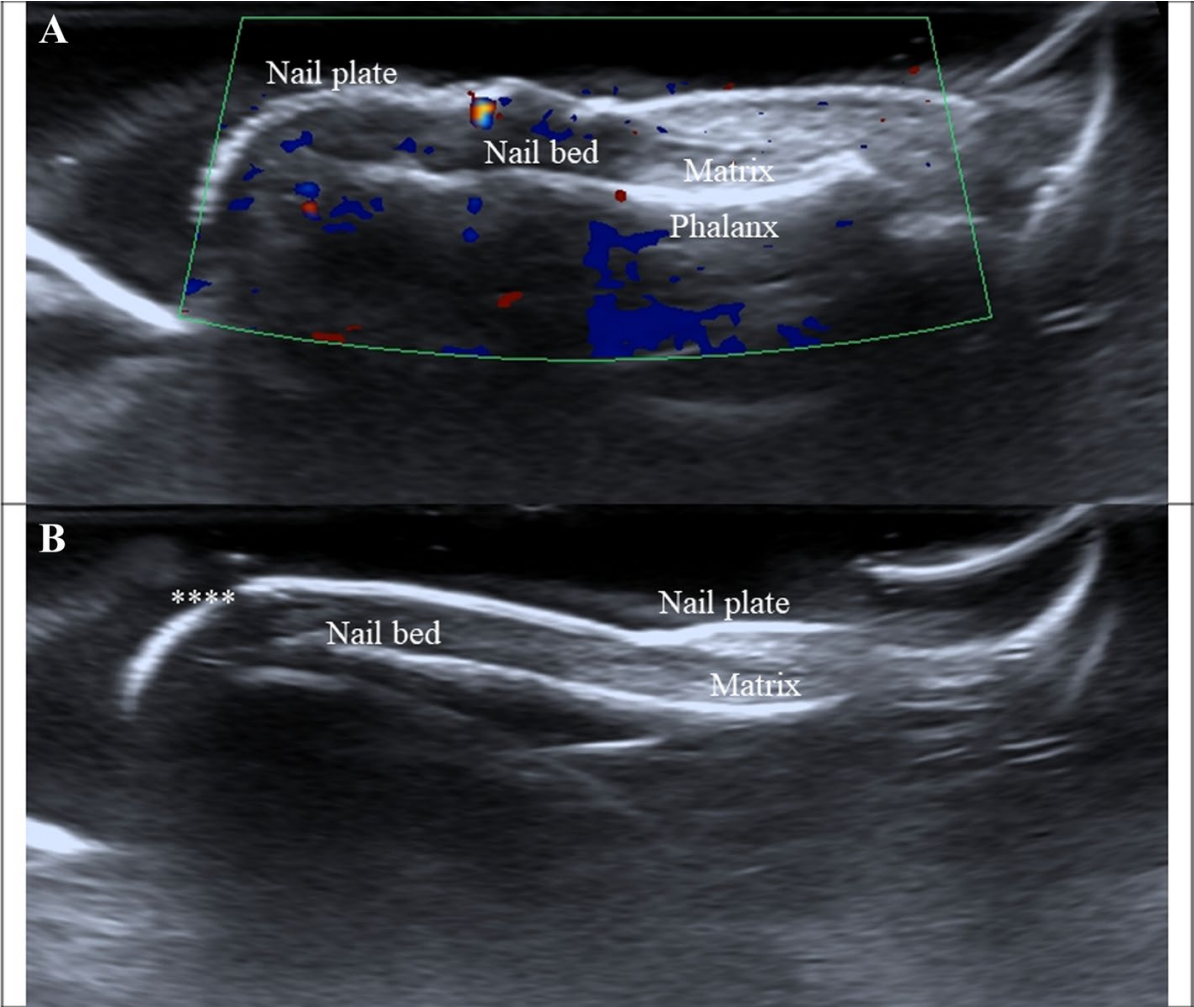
Nail ultrasonography. a Grayscale longitudinal plane ultrasonography and Doppler ultrasonography of the nail apparatus of the right hand fifth finger characteristic of phase III psoriatic onychopathy. The image shows wavy appearance with thickening of the nail plates, the nail bed shows focal hyperechoic involvement and thickening. The matrix does not have any changes. The nail vascularization is normal, as shown in the Doppler exploration. b Grayscale longitudinal plane ultrasonography and Doppler ultrasonography of the nail apparatus of the left hand fifth finger showing signs of early-stage phase I psoriatic onychopathy. The nail plates have normal morphology with hyperechogenic foci below the distal third of the ventral plate (). The nail bed, matrix, and distal interphalangeal joint are normal
